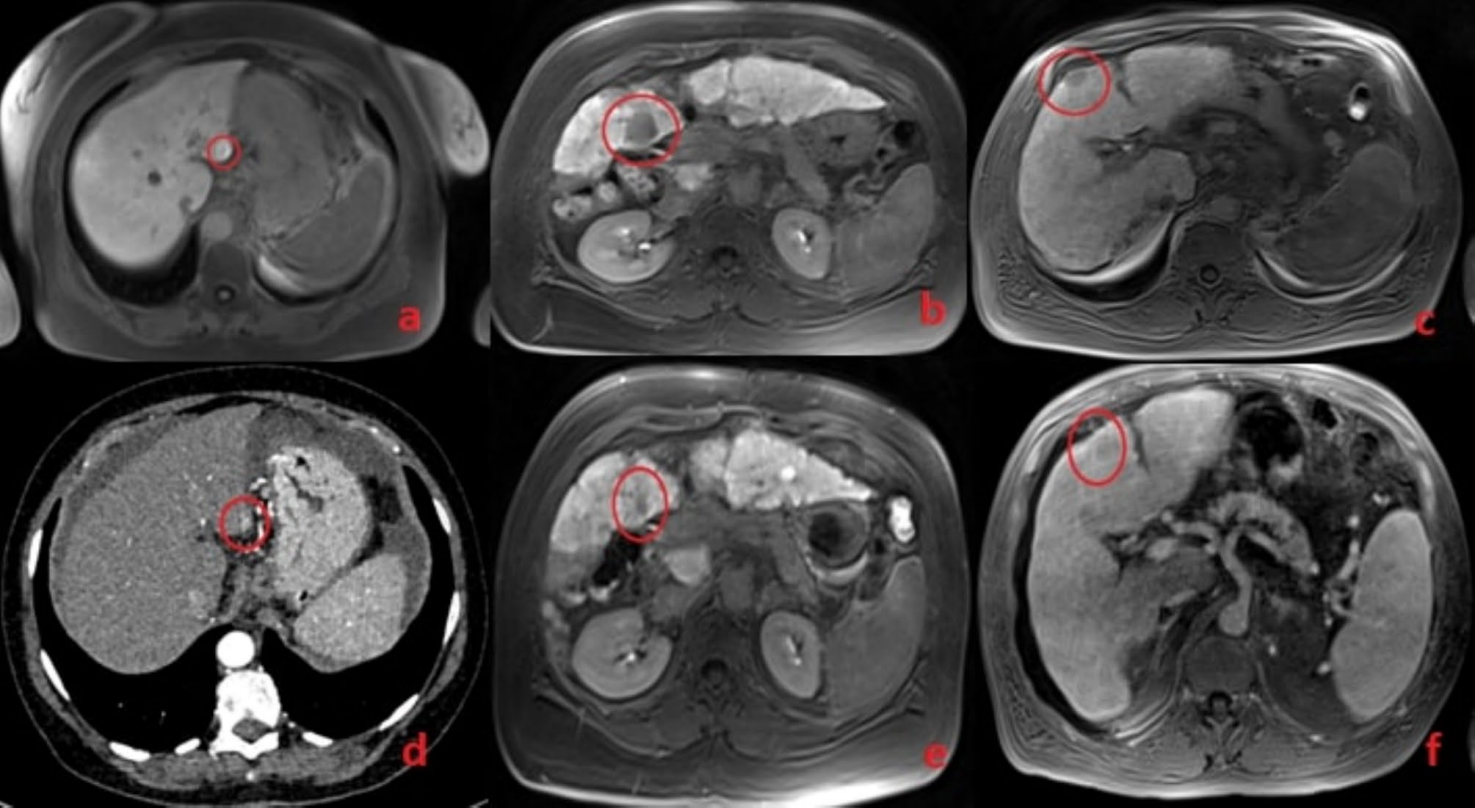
MRI and CT scans of LC patients at the time of NMR profiling (Images d, e, and f) and at the time of diagnosis (Images a, b, and c). The lower images (d, e, f) show potential precursor lesions that later developed into HCC at baseline, while the upper images (a and c) display the lesions identified as HCC during the 6-month follow-up. Image b shows the lesion identified at the 1-year follow-up
